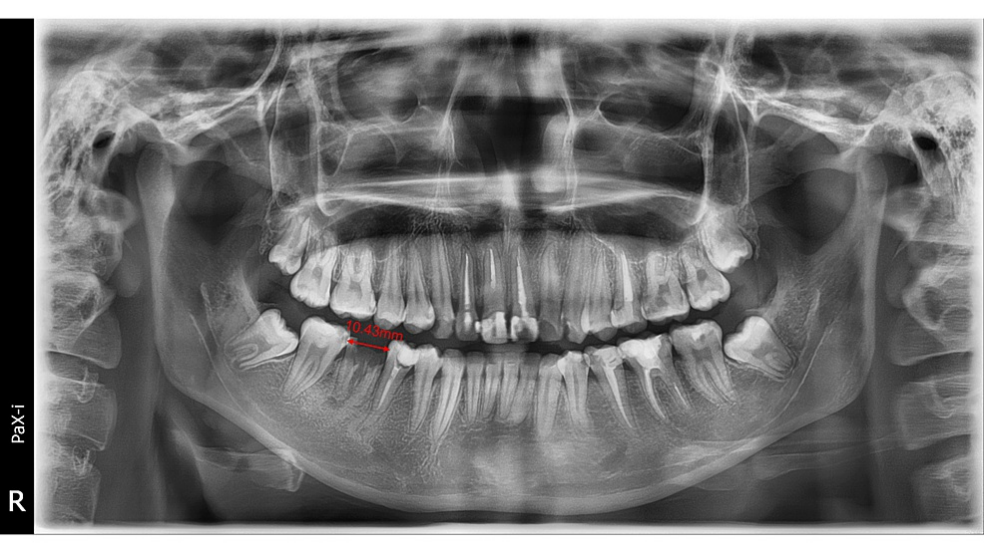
Pretreatment panoramic radiograph. It showed unrestorable #46 and the space between #47 and #45 was 10.43 mm.
